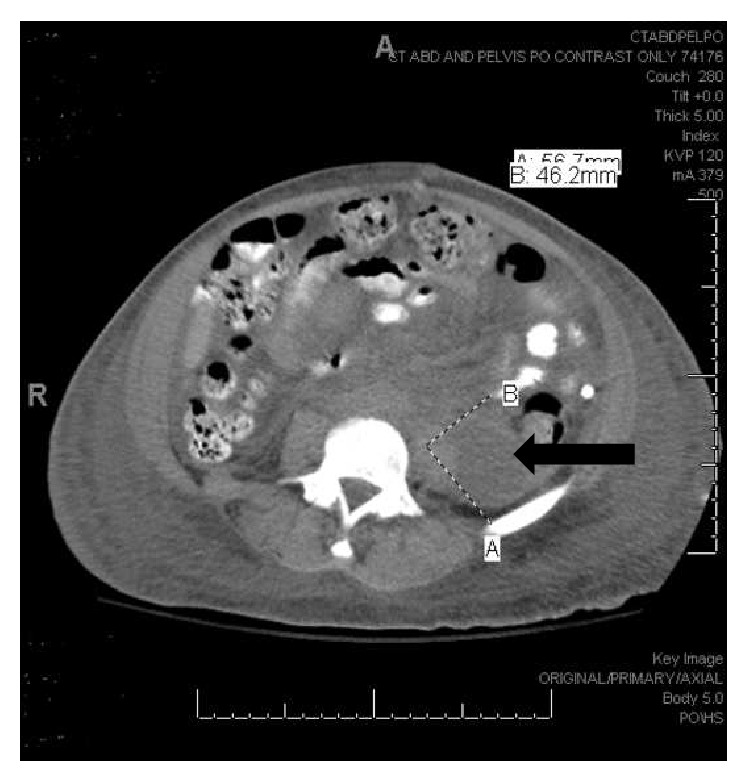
Repeat CT scan of the abdomen and pelvis with an ovoid fluid collection which measures 5.7 × 4.6 cm in the axial plane in the left posterior perinephric space.
